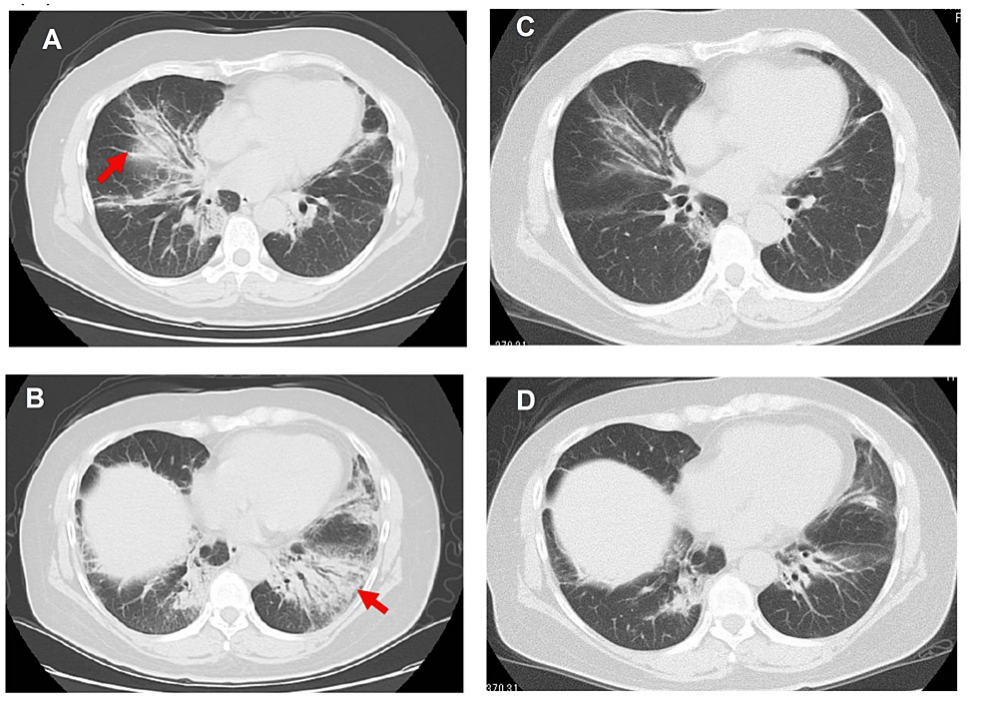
Chest CT of the patient before and after treatment. (A and B) Chest CT at the first visit: peribronchial infiltrative shadows are predominant in the bilateral lung bases; (C and D) Chest CT on day 19 of hospitalization: peribronchial infiltrative shadows are improved.
